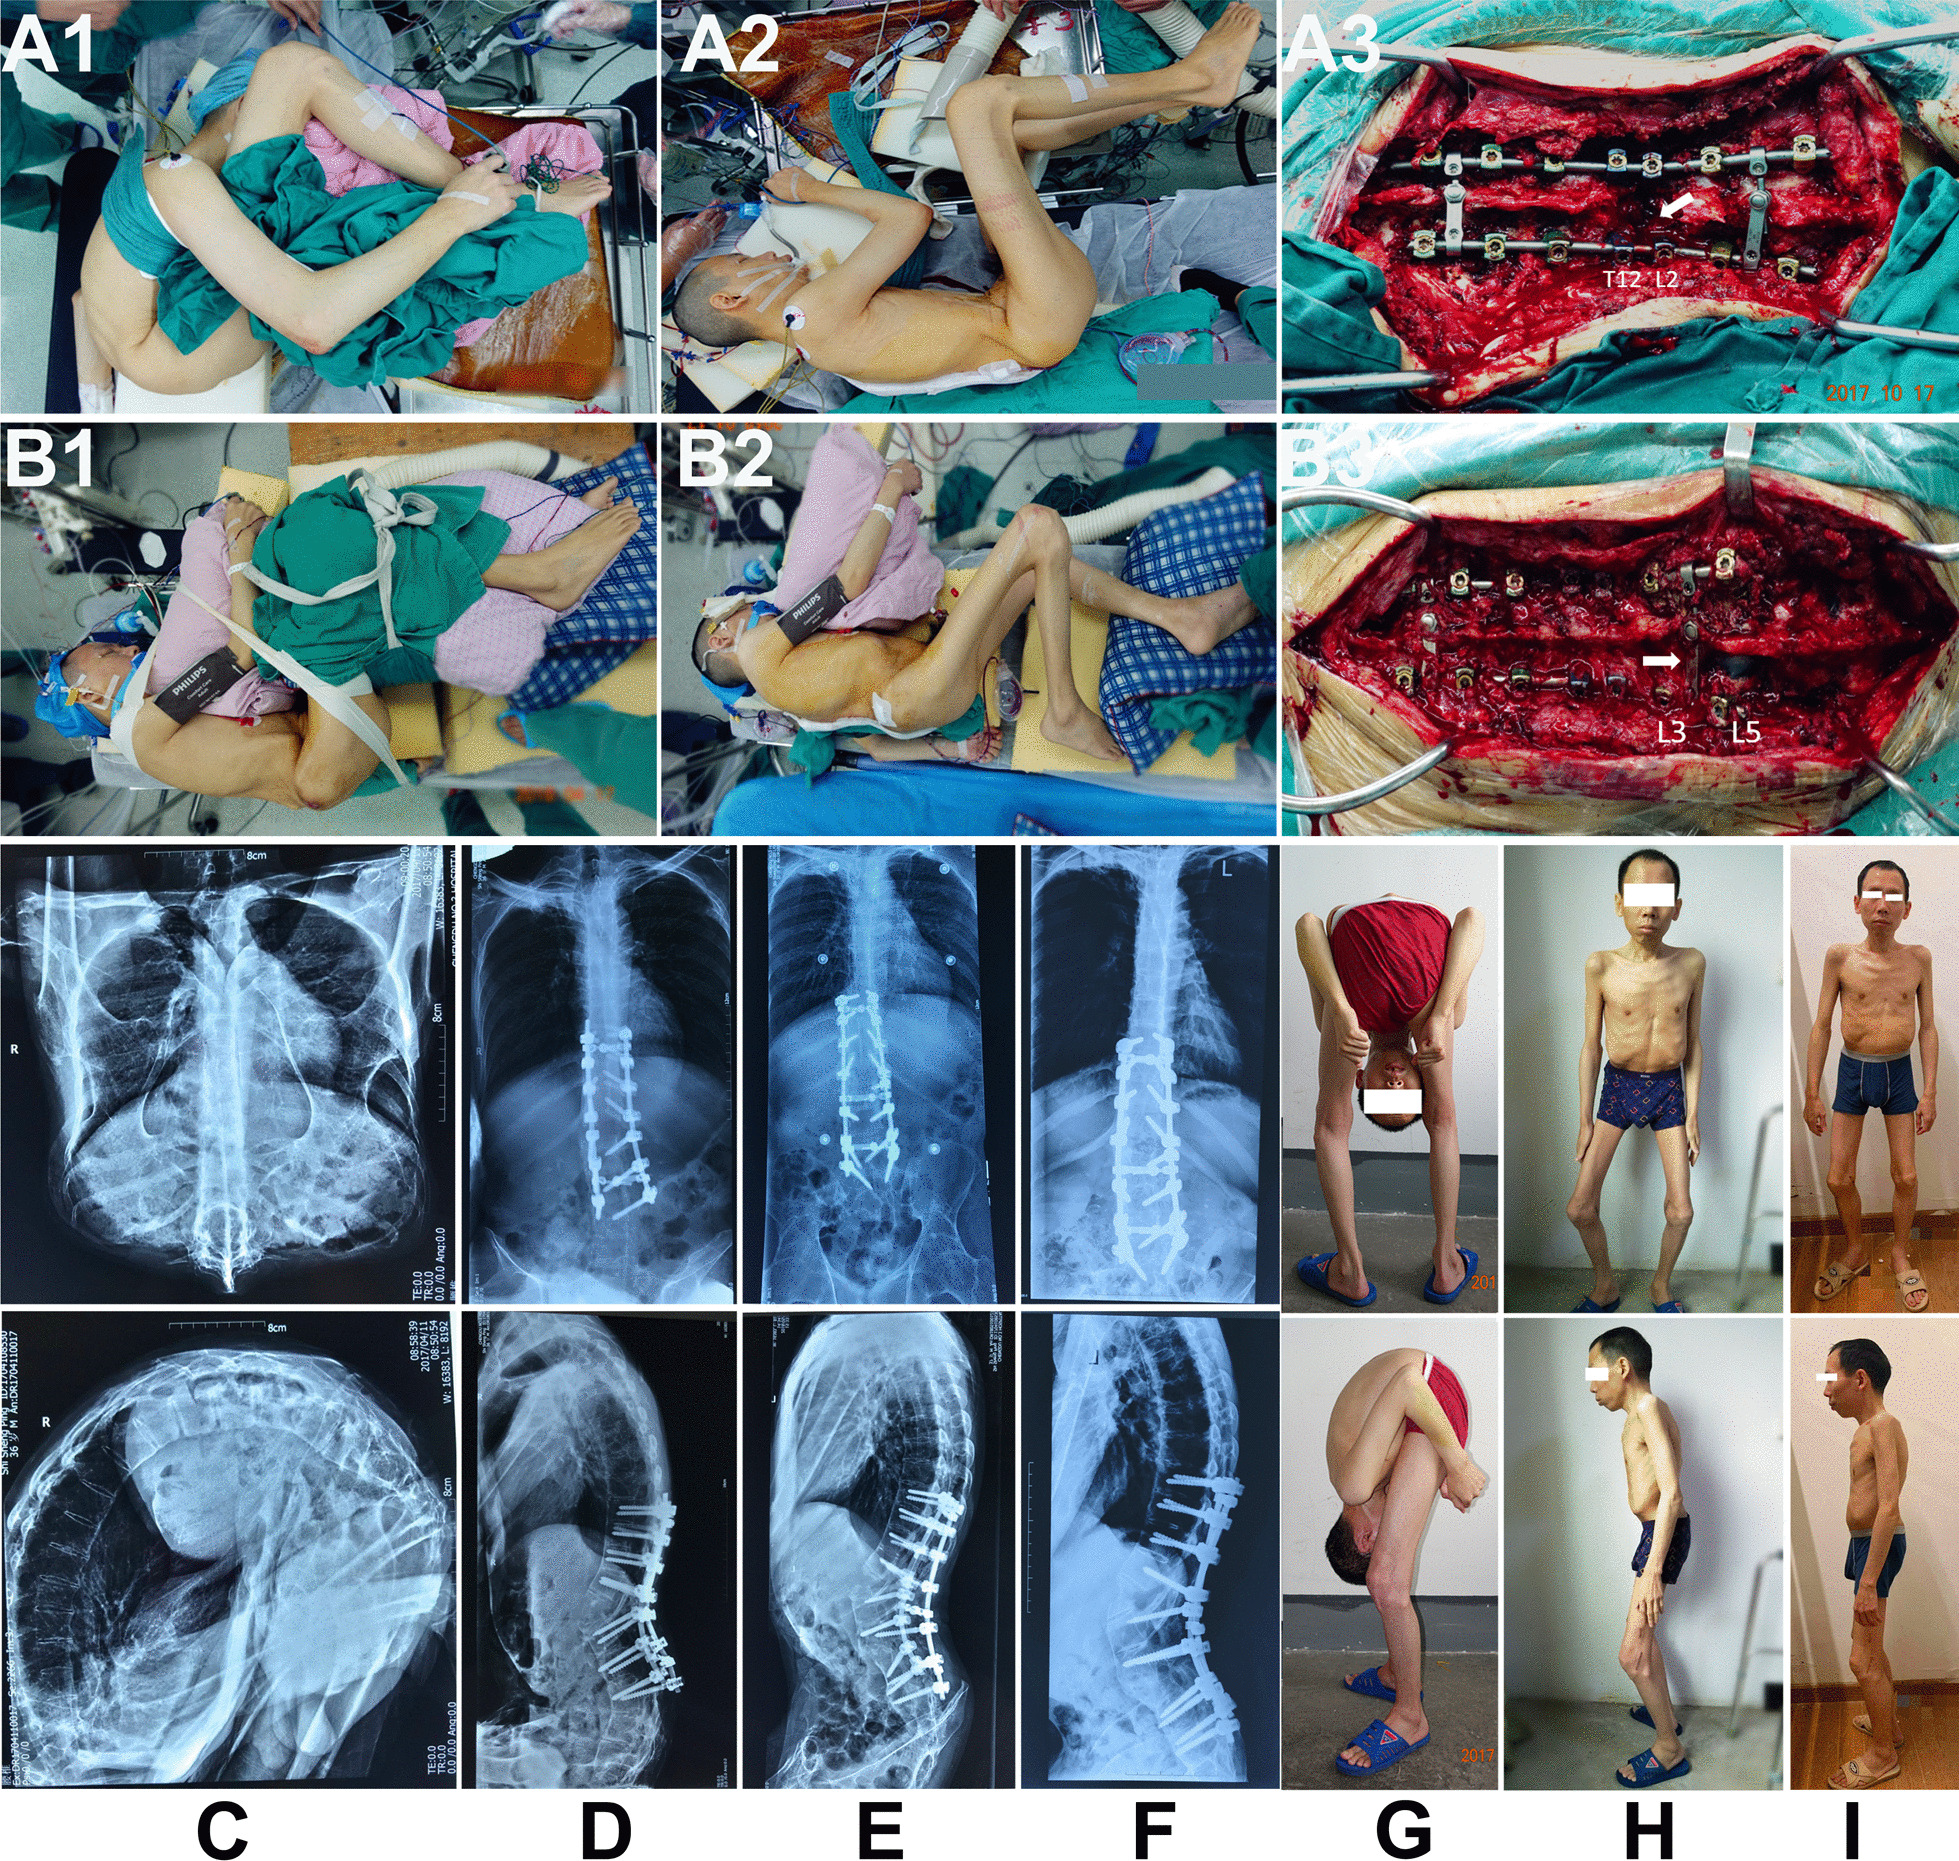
A 35-year-old man with a completely folded spine, who cannot undergo corrective surgery in a prone position. A1–A3 display the first-stage L1 PSO in lateral position; B1–B3 exhibit the second-stage L4 PSO in lateral position. C–F present preoperative, post-first-stage osteotomy, post-second-stage osteotomy and final follow-up radiographs. G–I depict preoperative, immediate postoperative and 31 months postoperative appearances. White arrows indicate osteotomy level
